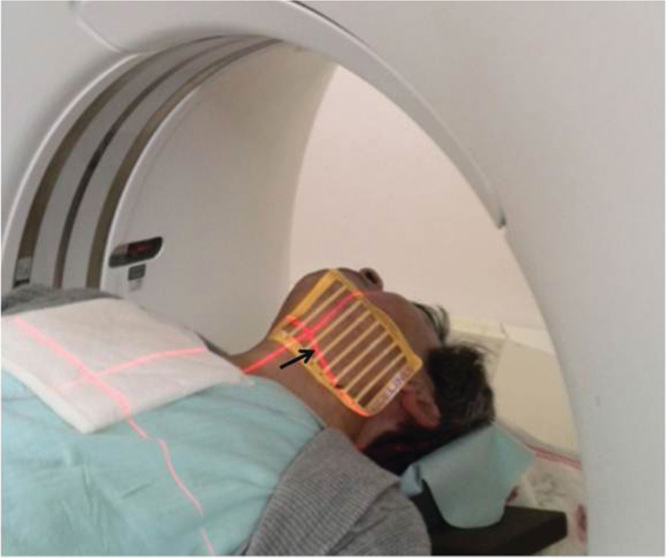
The sentinel lymph node (SLN) location was indicated precisely by the crossing points of the lattice marker and the CT plane light. Arrow, CT plane light.
